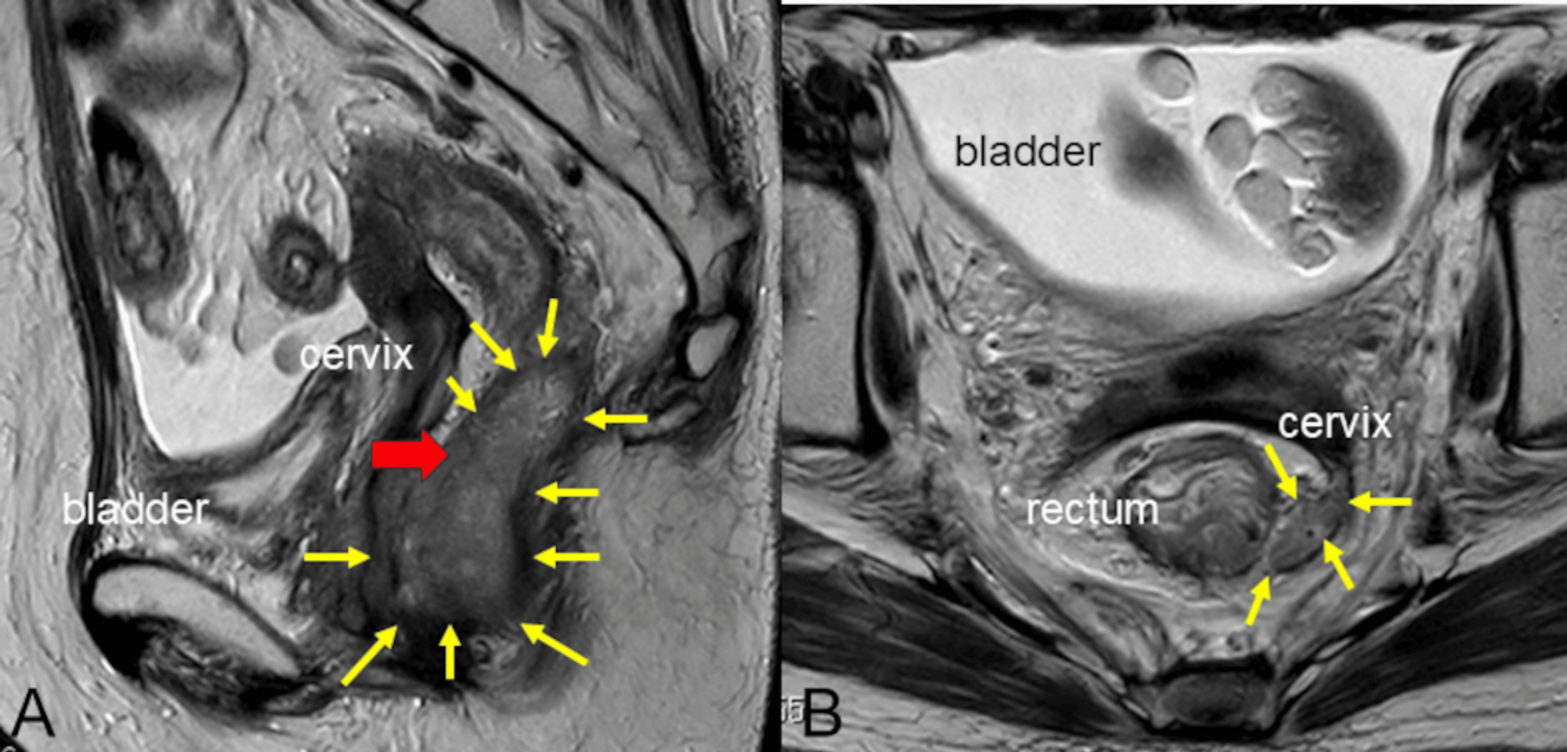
MRI images five years after CCRT A: Sagittal section; B: Horizontal section; Yellow arrow: tumor; Red arrow: biopsy point CCRT: concurrent chemoradiotherapy
